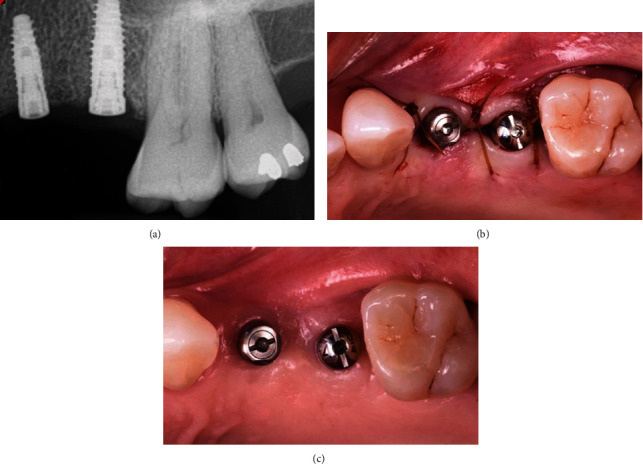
(a) At three months postimplant placement, the radiograph showed slight crestal bone loss. (b) Implants exposure with healing abutments. (c) Two weeks healing with better soft tissue healing and healthier-looking tissue.
